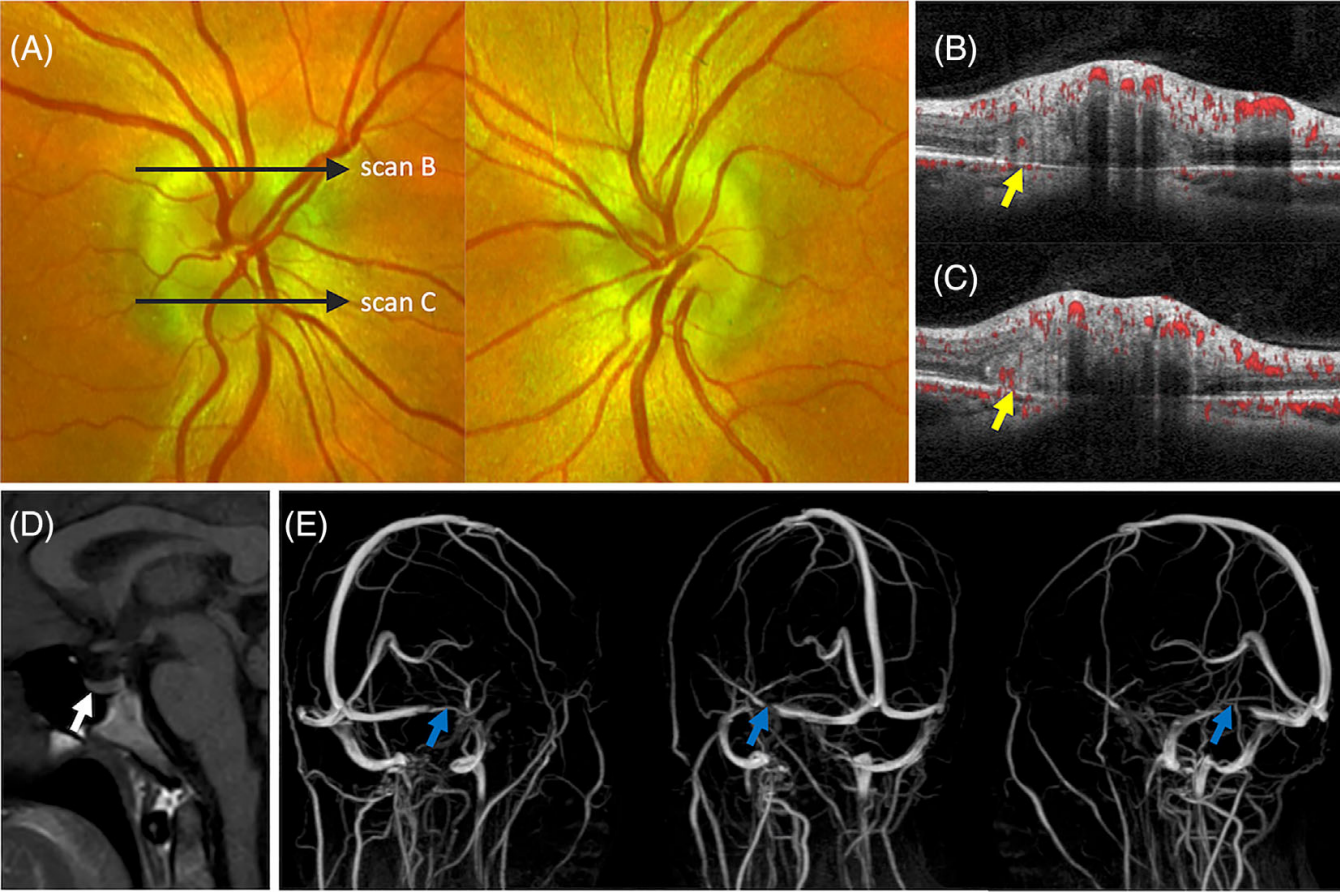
A 45‐year‐old obese female with idiopathic intracranial hypertension presented with headache, bilateral visual loss and papilloedema (A). The opening pressure was 27 cm water. OCT showed hyperreflective PHOMS with internal flow signals (yellow arrows) at the superior (B) and inferior (C) disc margins. Magnetic resonance imaging and venogram showed patulous optic sheath complexes, partially empty sella (white arrow) configuration (D) and bilateral flow gaps around the lateral angles of both transverse sinuses (blue arrows) indicating relative stenosis (E).
